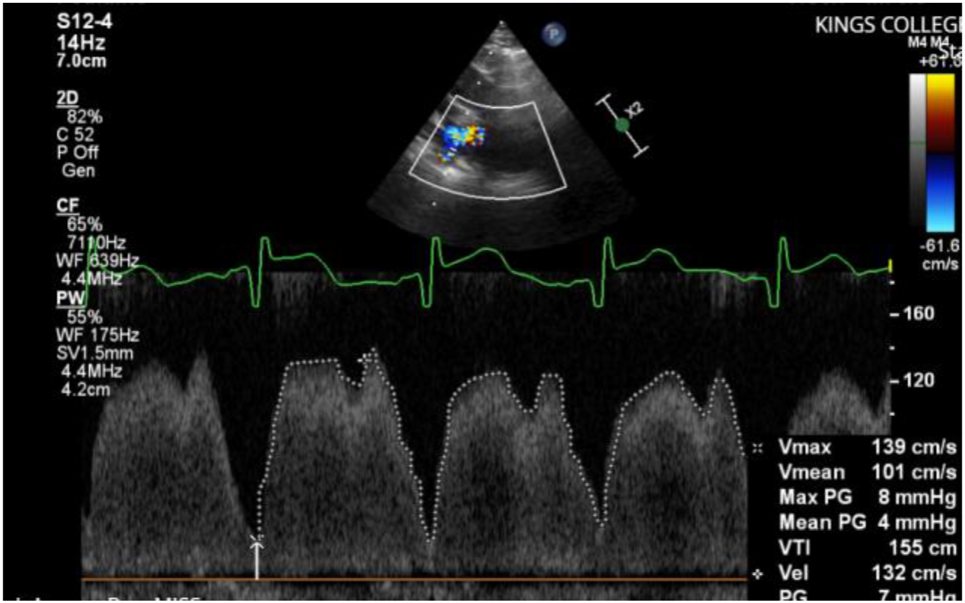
Post dilatation. 2D echocardiography and pulse wave Doppler of pulmonary vein demonstrating reduced velocity (1.39 m/s) and mean pressure gradient (4 mmHg) post dilatation. Note that the pulse waveform does return to baseline, in keeping with an unobstructed pulmonary vein.
